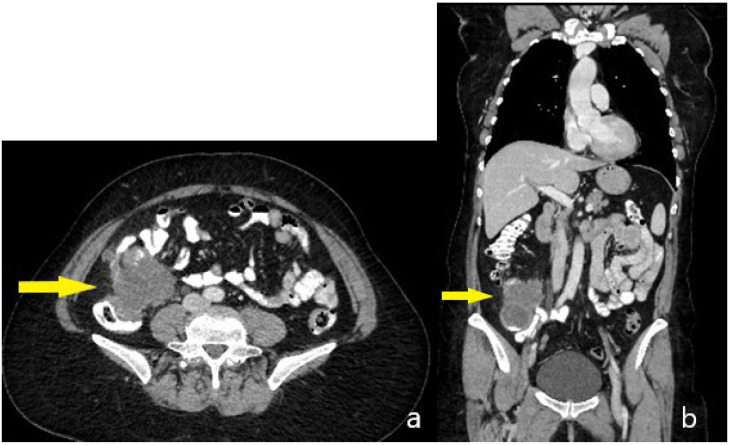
Preoperative CT scan—(a) Transversal aspect, tumor–arrow, (b) Sagittal aspect, tumor–arrow, no distant visible metastases.
